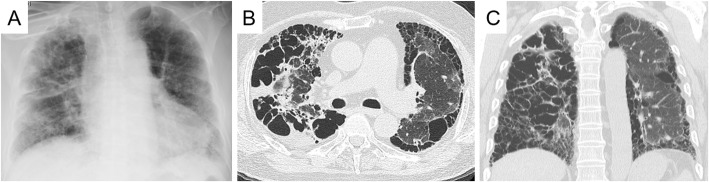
a) Preoperative chest X-ray and b), c) chest computed tomographic images of the recipient. The preoperative chest X-ray and chest computed tomographic images revealed heterogeneous disease progression, with predominant damage in the right lung
